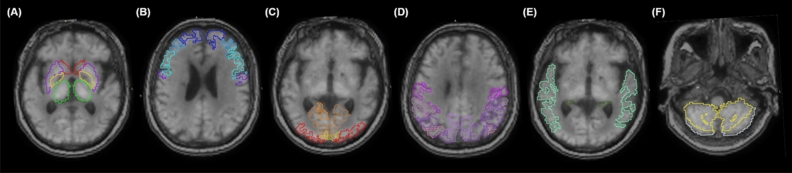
Representative images for outline contours of volumes of interest (VOIs). The VOIs for central structures (A), frontal (B), occipital (C), parietal (D), temporal (E) lobes, and cerebellum (F) with colored outline contours were automatically set in co-registered PET-MR images by the AAL-merged atlas provided by PMOD.
